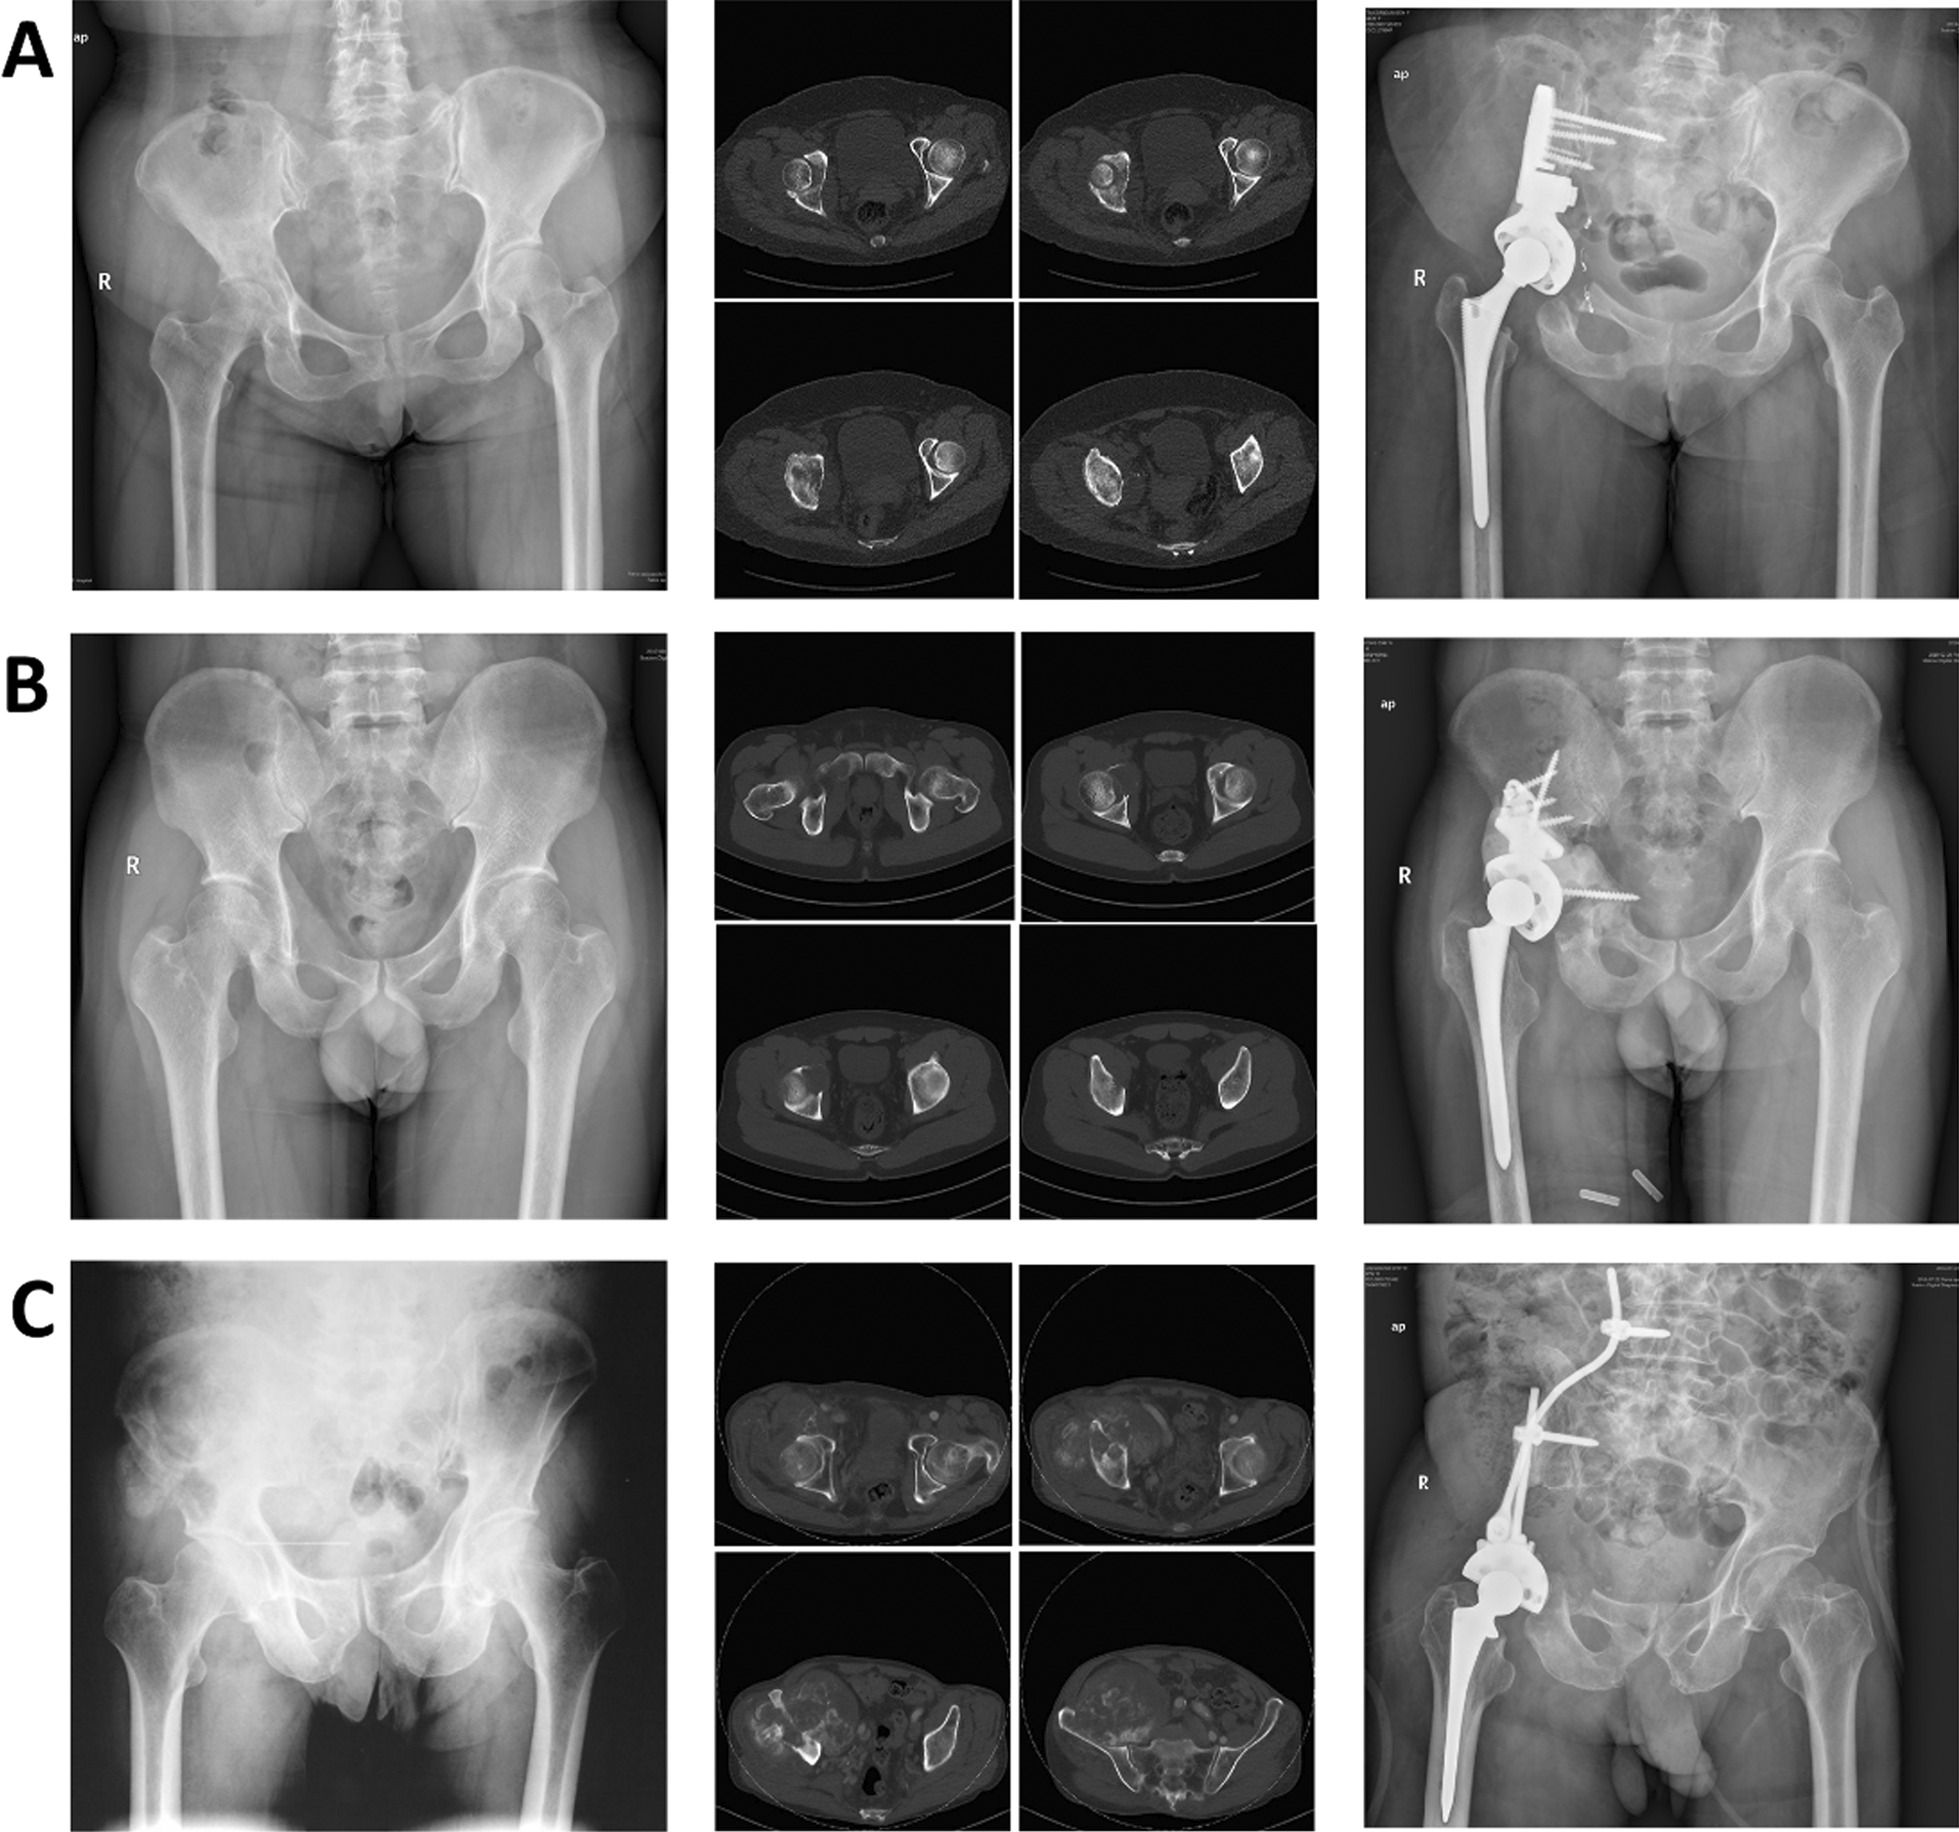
Typical cases of three kinds of hemipelvic prostheses. A Radiographs of a 53-year-old woman showing chondrosarcoma of the right pelvis involving acetabulum and ilium (Case 4 in Table1). B Radiographs of a 33-year-old man showing a malignant giant cell tumor of the right pelvis involving the acetabulum (Case 7 in Table1). C Radiographs of a 75-year-old man showing dedifferentiated chondrosarcoma of the right pelvis involving acetabulum and ilium (Case 20 in Table1)
